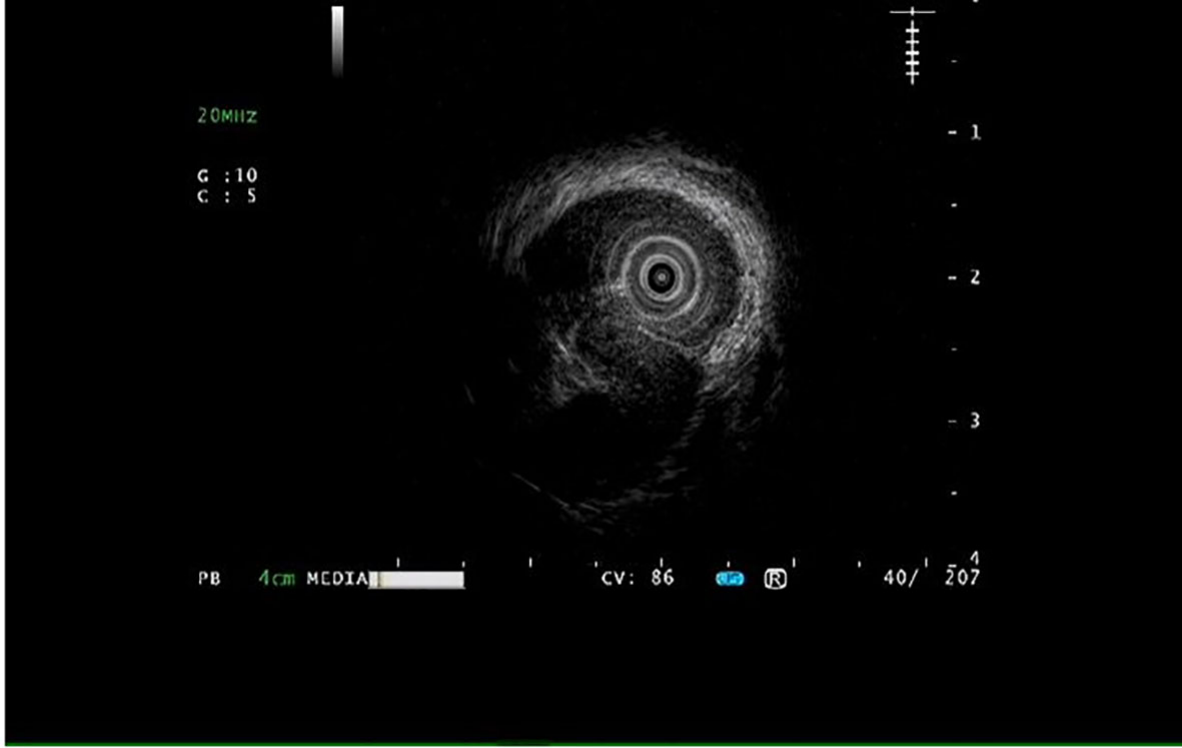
Endoscopic ultrasonography reveals that the structures of the first, second, and third layers are clear and intact, with a homogeneous hypoechoic area observed in the fourth layer.
